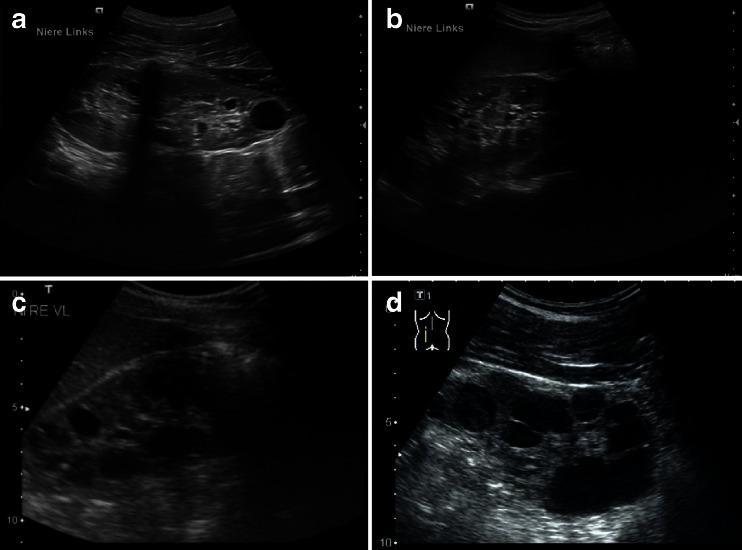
Typical sonographic images of autosomal dominant polycystic kidney disease (ADPKD) in different patients of adolescent age
